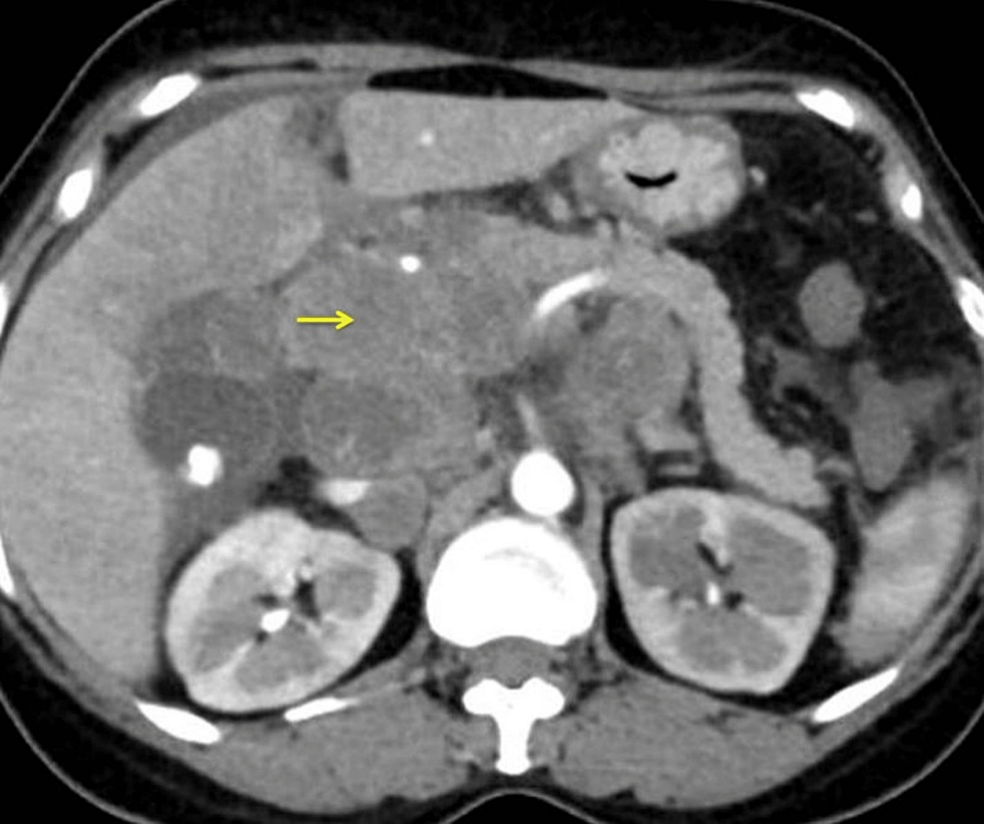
CT of the abdomen showing conglomerated lymph nodal mass near the celiac axis (arrow)
